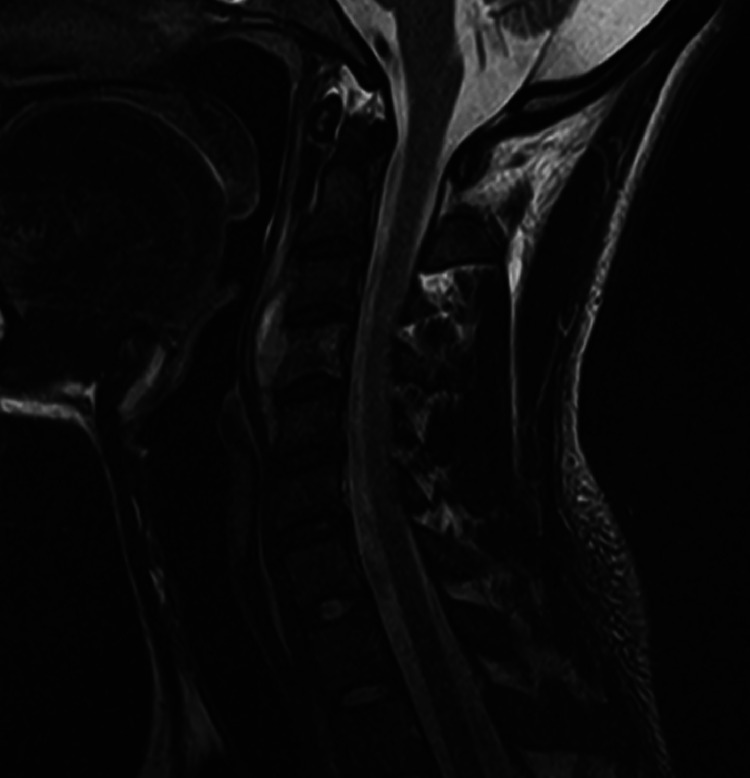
MRI of the cervical spine. Enhancing soft tissue noted epicentered along the anterior margin of C4 vertebral body with loss of anterior cortex and mild marrow enhancement, extending both superiorly and inferiorly along the C3 and C5 vertebra. Findings suggest subligamentous spread of TB, characterised by relative sparing of the disc spaces.
